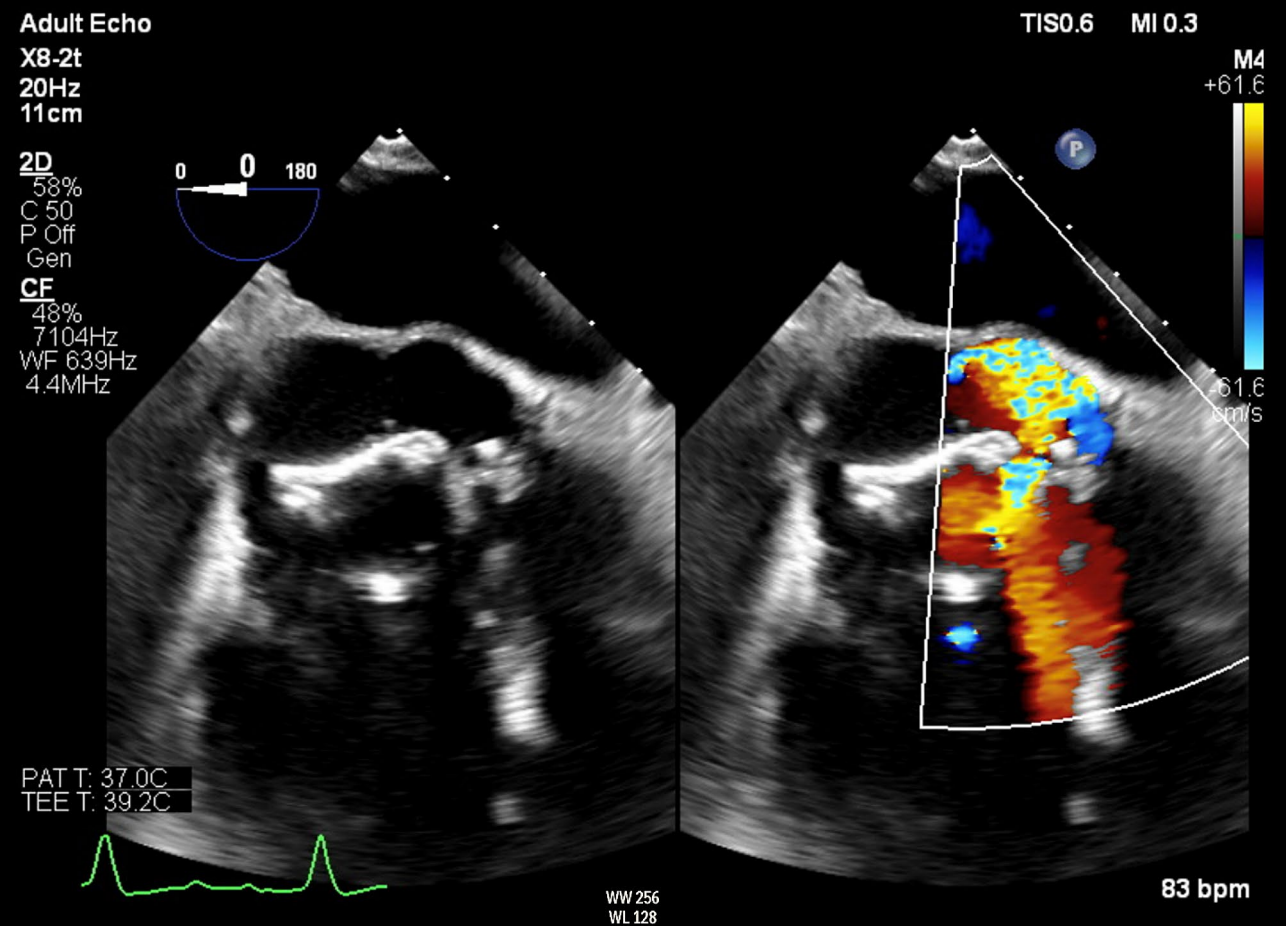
Transoesophageal echocardiogram short axis view of the rocking aortic valve
